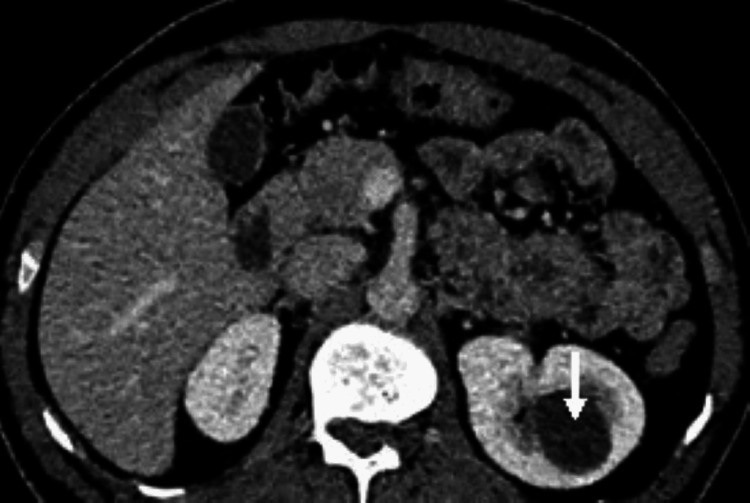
CT nephrographic phase, shows a cystic lesion at the upper pole corticomedullary junction.
